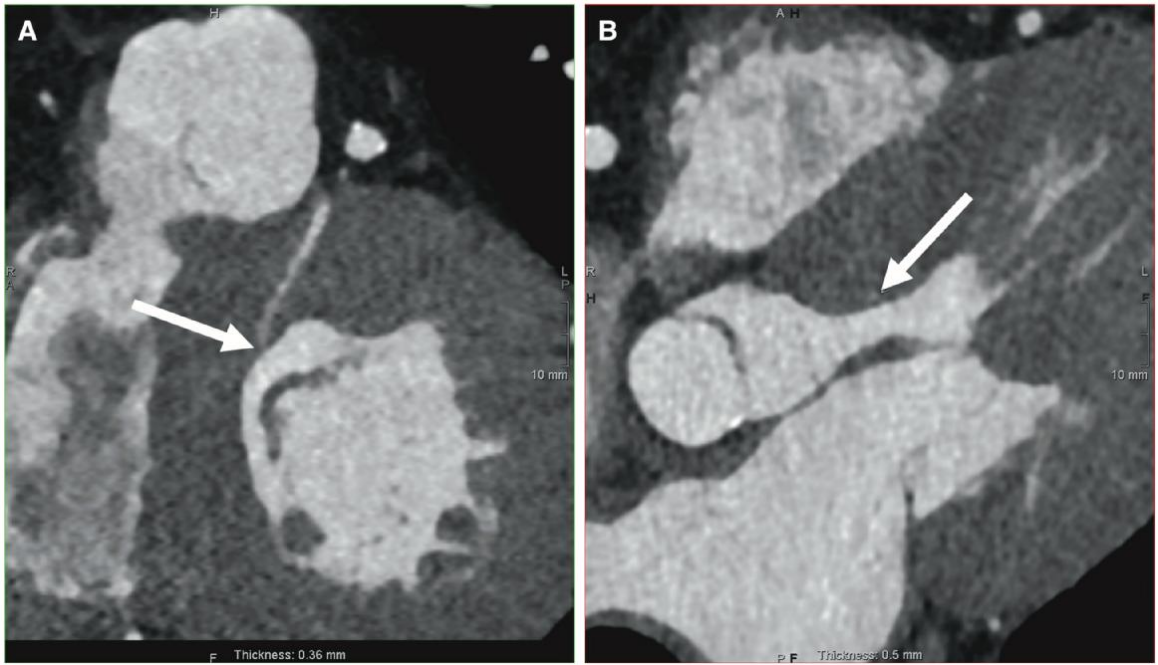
Computed tomography coronary angiography demonstrating the course of first septal perforator from the left anterior descending artery. Although the course of the perforator was determined by multiplanar reconstruction (*A*), its course into the left ventricular cavity could only be suggested (*B*).
